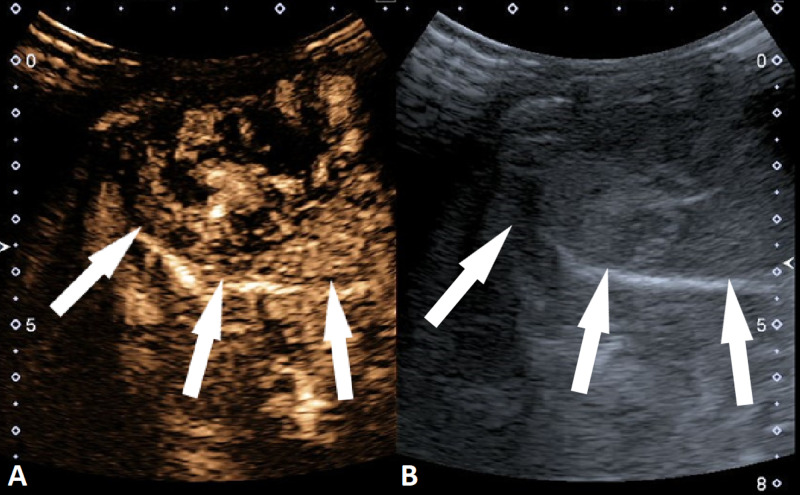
Abdominal contrast-enhanced ultrasound (CEUS) (A) An abdominal CEUS showing peripheral nodular enhancement of the lesion (white arrows) eight seconds after IV contrast administration; (B) parallel to the CEUS, a gray-scale US of the lesion (white arrows).
